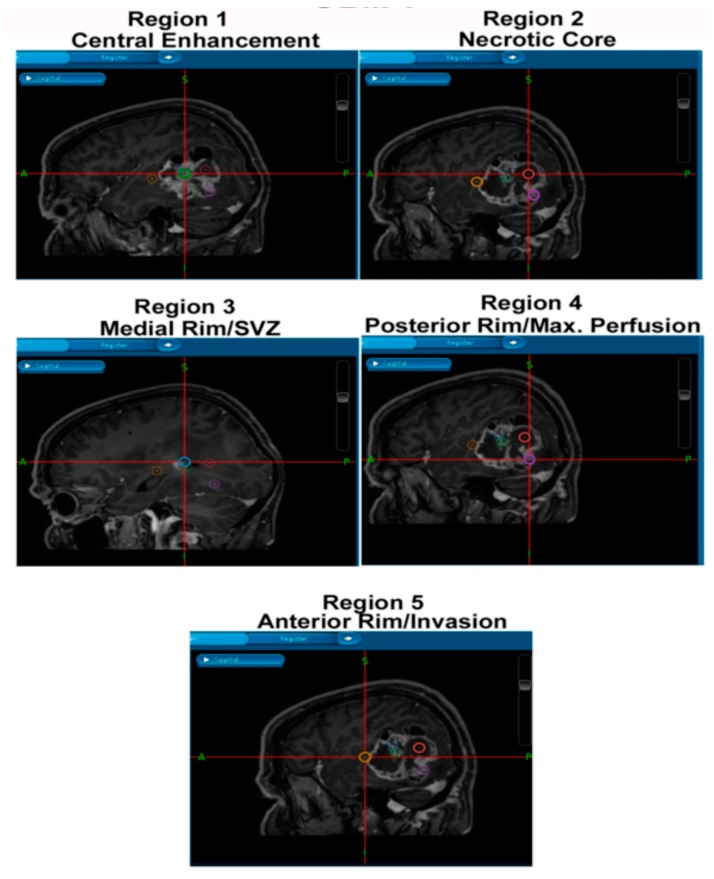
Multi-regional surgical sampling of primary glioblastoma multiforme (GBM). T1-weighted MRI scans of a representative patient, with cross-hairs depicting spatially-distinct multi-region surgical sampling. Regions include central enhancing core, necrotic core, medial and posterior rims and invasive margin (Region 5).
